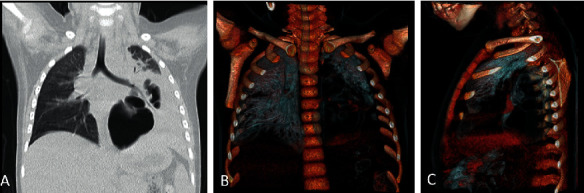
Noncontrast frontal view chest CT: lung window (a) and virtual bronchography (volume-rendered display) for visualization of airways (b, c).
